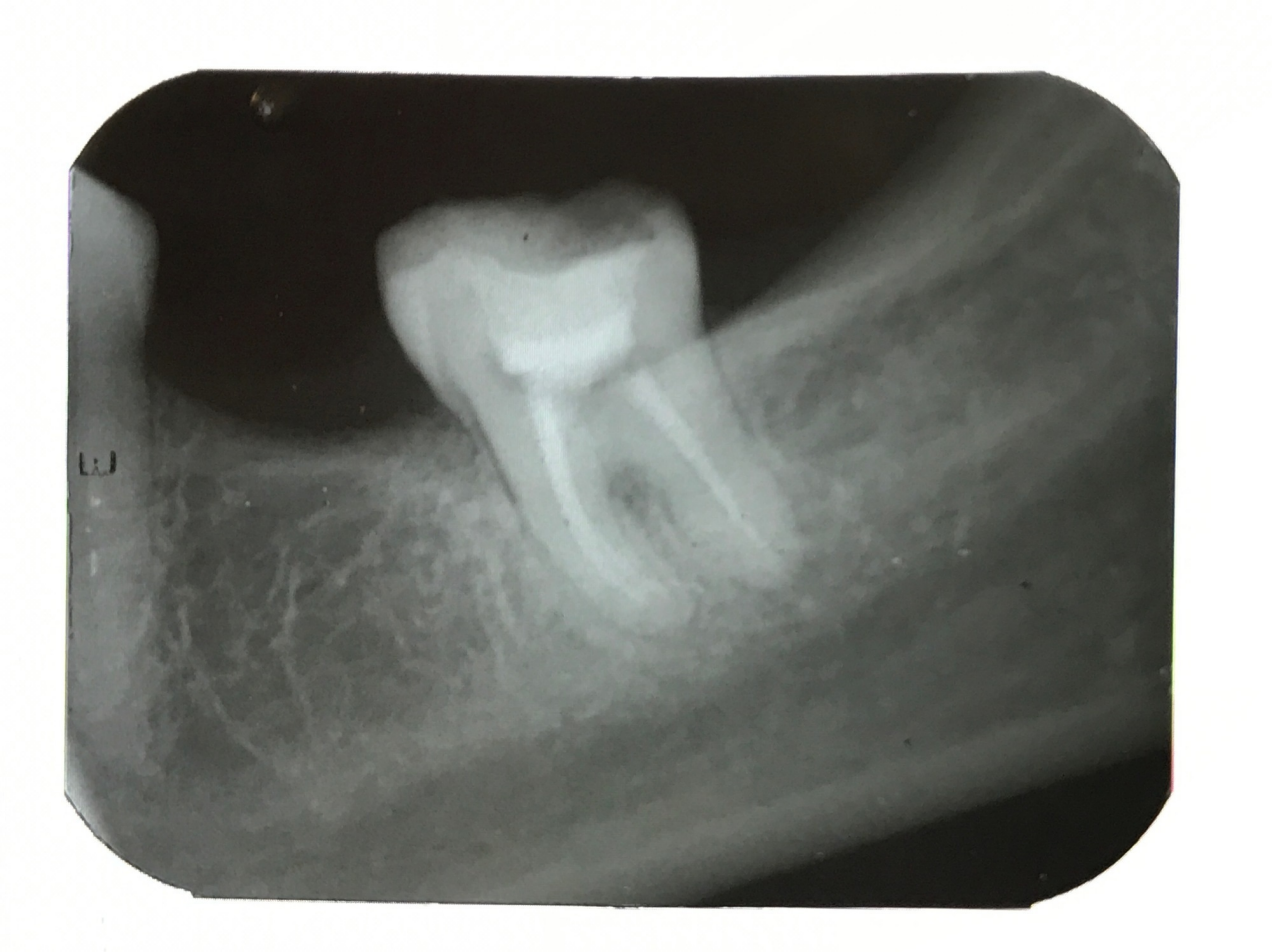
Postoperative Intraoral Radiograph
